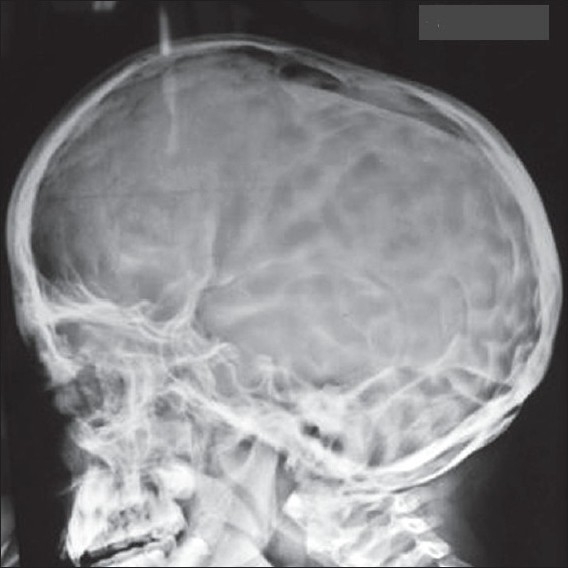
Skull X-ray showing osteopenic lesions and retained primary dentition
